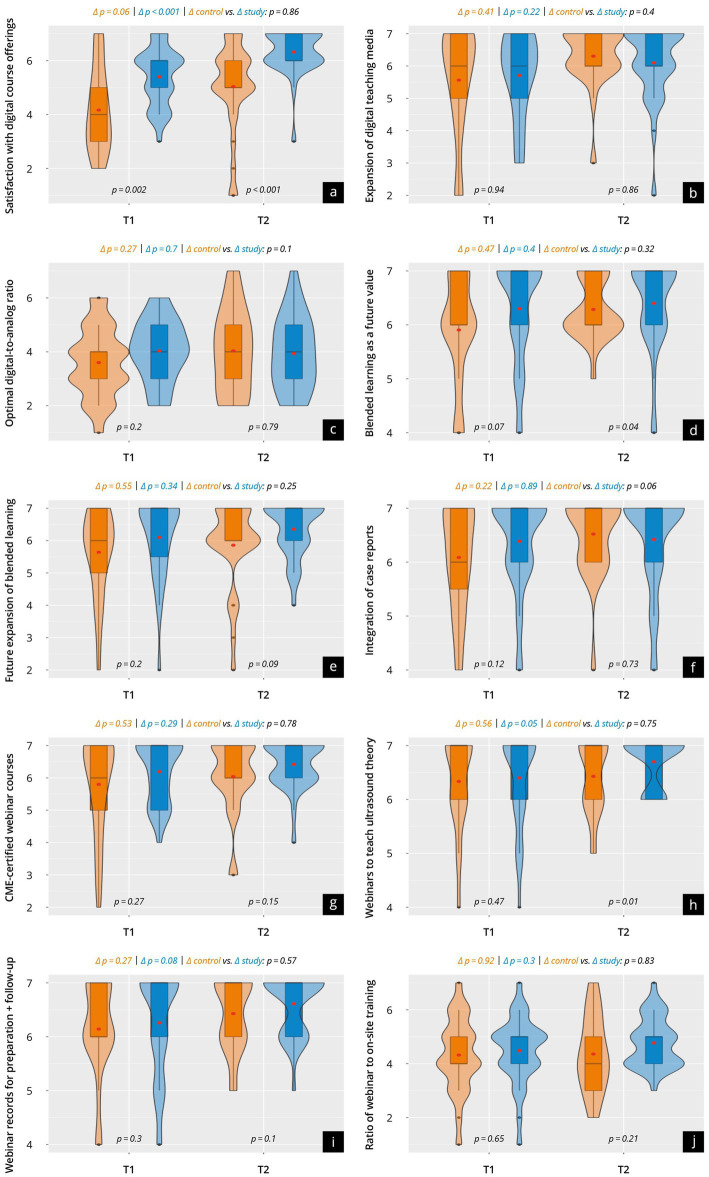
Results of the evaluation of attitudes toward digital training concepts and prospects in a group comparison at time points T1 and T2. The violin plots present the results of the following surveyed items: (a) Satisfaction with digital course offerings; (b) Expansion of digital teaching media; (c) Optimal digital-to-analog ratio; (d) Blended learning as a future value; (e) Future expansion of blended learning; (f) Integration of case reports; (g) CME-certified webinar courses; (h) Webinars to teach ultrasound theory; (i) Webinar records for preparation and follow-up; (j) Ratio of webinar to on-site training.
